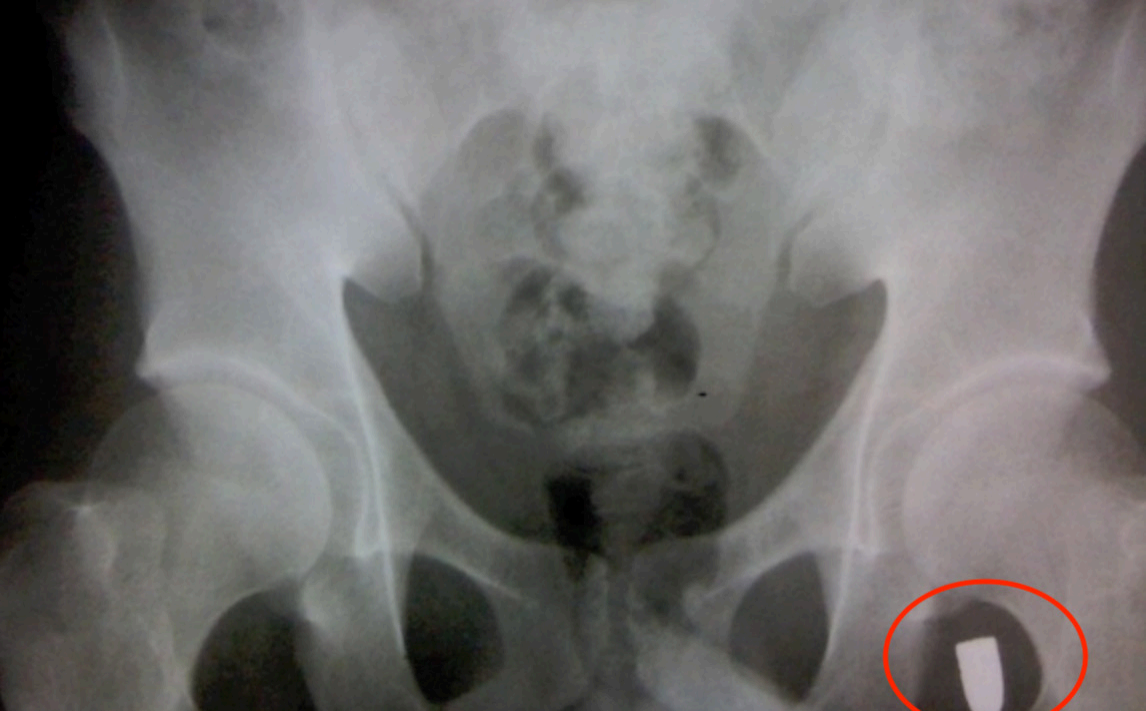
Abdominal x-ray. Red circle showing the bullet in the left inguinal region
